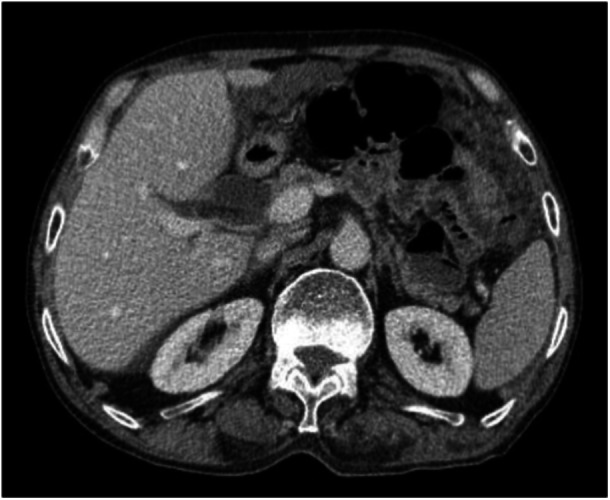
Nodularity of the peritoneum in CT scan. CT, computed tomography.
